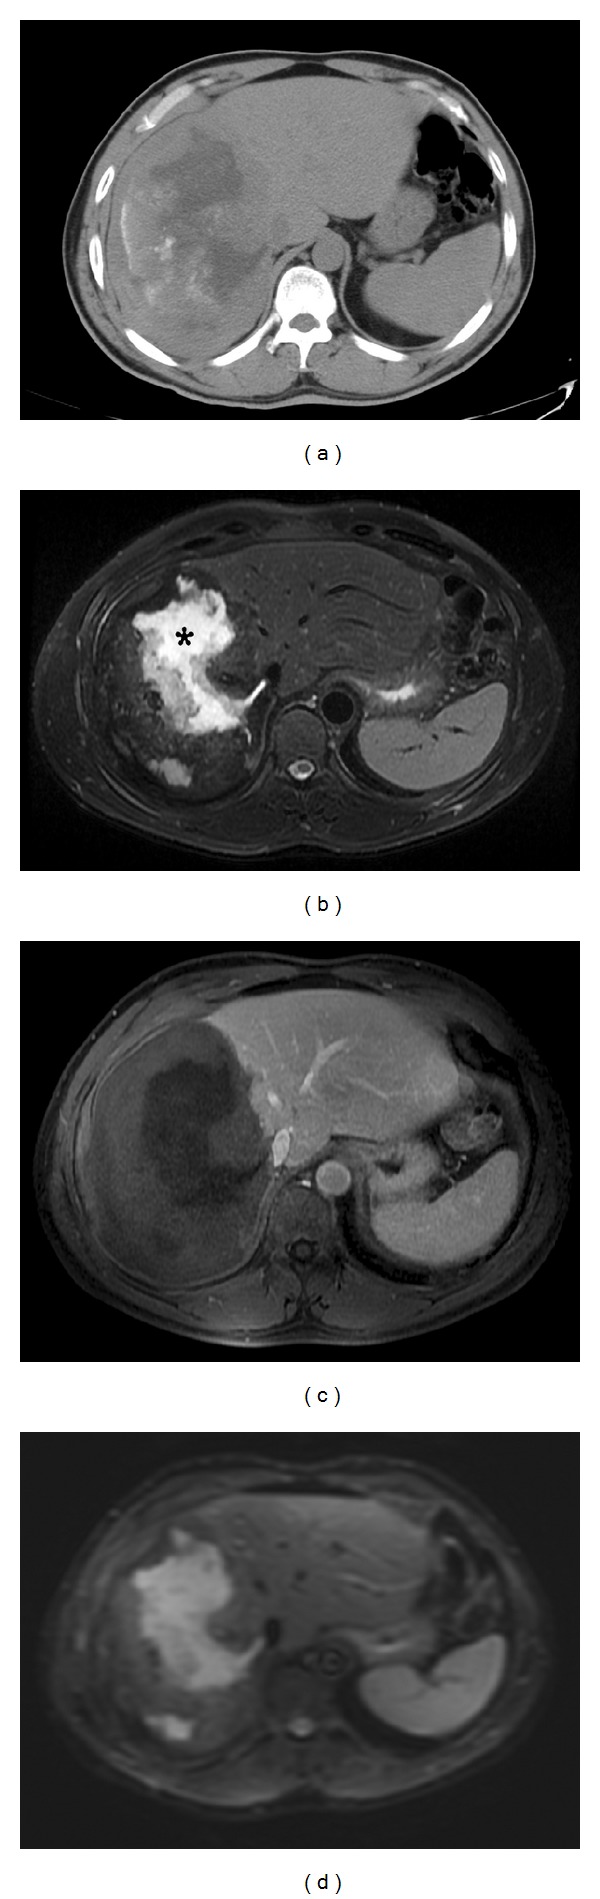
Unenhanced CT scan shows an infiltrating hepatic solid mass that contains multiple cystic-necrotic hypodense areas and hyperattenuating foci of internal calcifications (a). Necrotic areas (asterisk) of the mass are clearly seen in an axial T2-weighted fast recovery fast spin echo (FRFSE) image (b). Gadolinium-enhanced T1-weighted gradient-echo, dynamic 3D-liver acquisition with volume acceleration (LAVA) image depicts no contrast enhancement in the lesion (c). Relative to the surrounding liver parenchyma, the central areas of the mass remain hyperintense on the diffusion-weighted (*b* value = 500 s/mm^2^) spin echo-echo planar imaging (SE-EPI) image (d).
